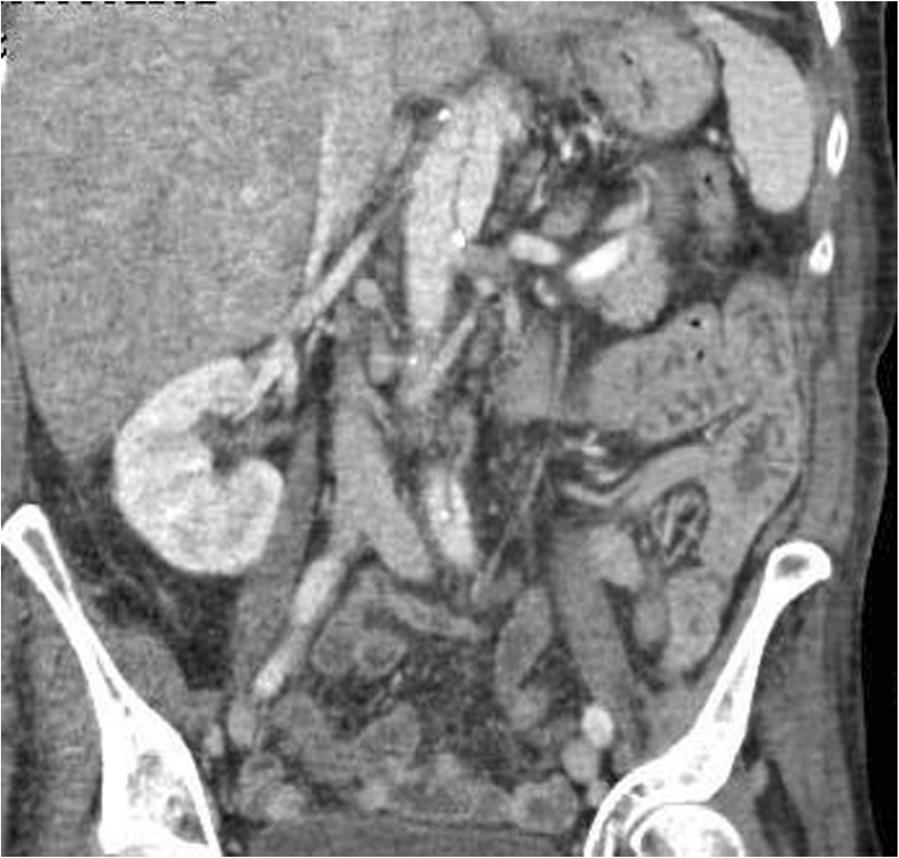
CT scan showing chronic upper abdominal aortic dissection.
